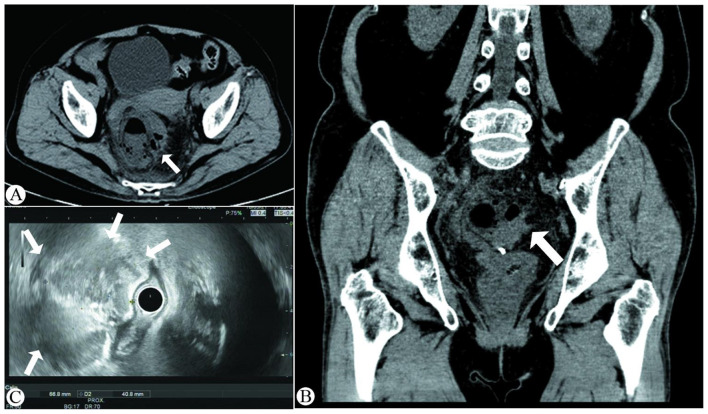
Post-EMR imaging findings of the patients. (A, B) CT scans demonstrating rectal wall thickening, associated with intramural gas and blurred perirectal fat spaces (white arrow). (C) EUS (7.5 MHz) revealing a hypoechoic lesion arising from the second layer of the rectal wall (white arrow).
